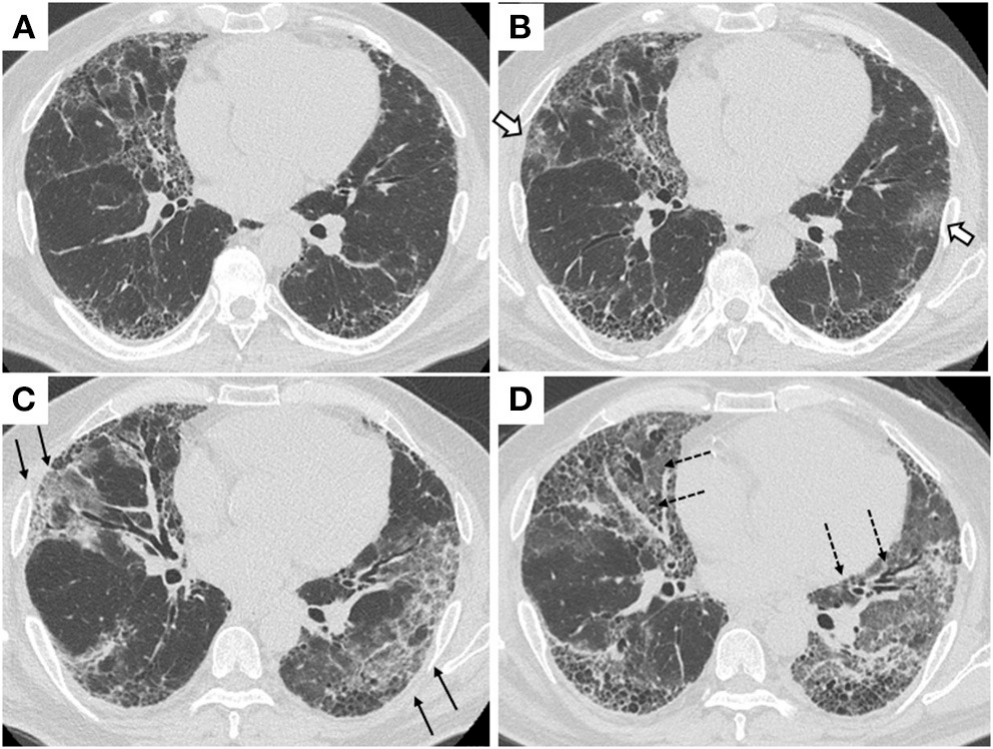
Chest CT scans. (A) On his last regular visit prior to the first admission. (B) On his first admission due to COVID-19. Open arrows indicate newly developed ground-glass opacities in the outer zones of both lungs. (C) On day 15 of his first admission, just before discharge. Arrows indicate ground-glass opacities were increased but limited to the outer zones of both lungs. (D) On his re-admission due to AE-IPF. Broken arrows indicate extensive ground-glass opacities spread to the inner zones of both lungs.
